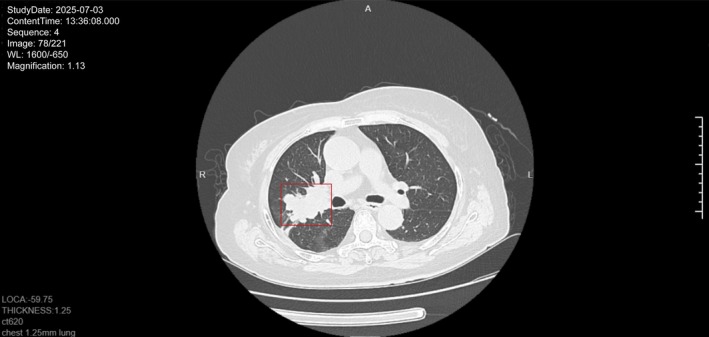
Chest computed tomography performed after admission revealed an irregular mass (approximately 47 × 30 mm) adjacent to the hilum of the right upper lobe.
